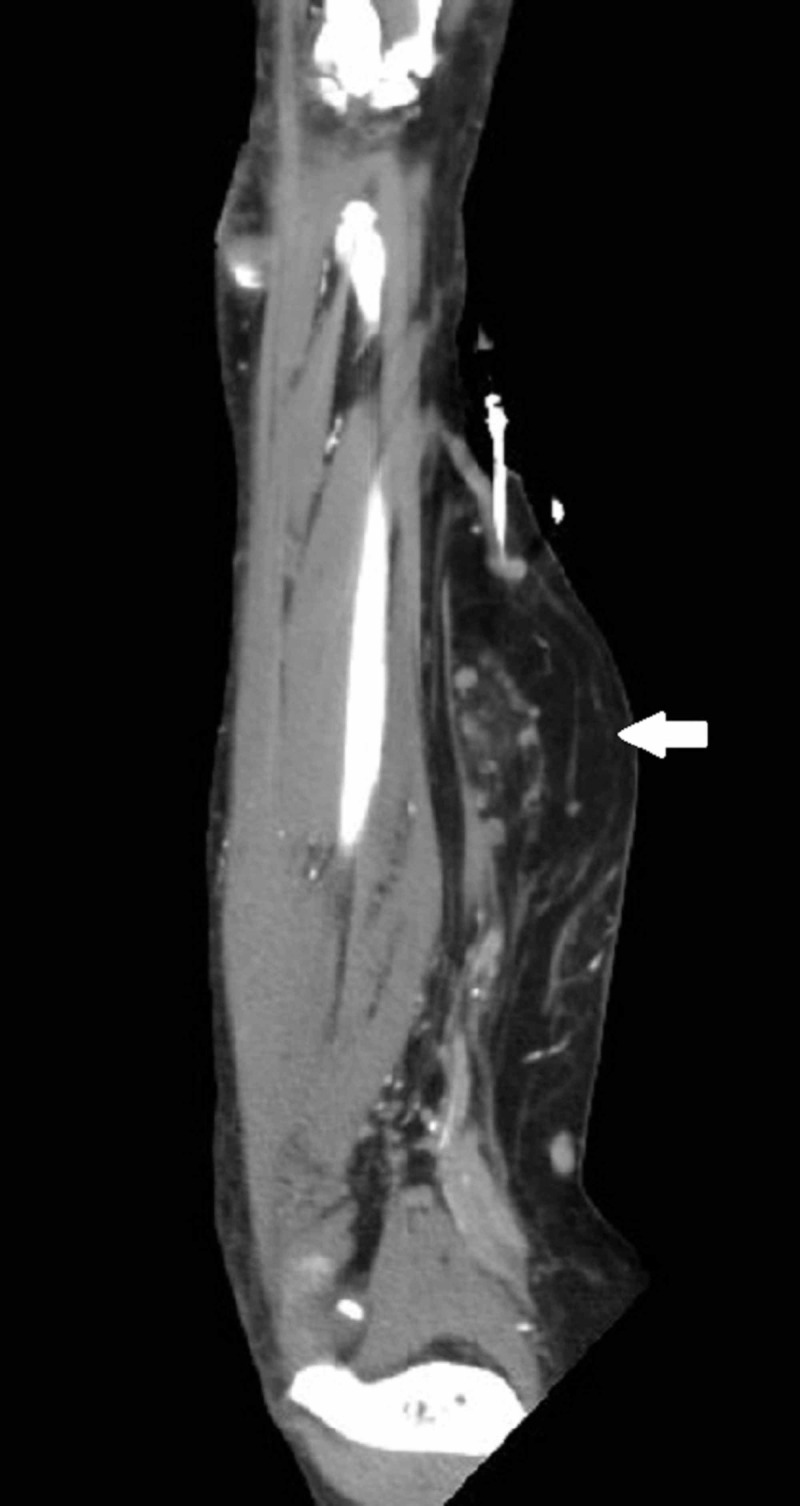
Coronal reformatted CT also showing a right forearm mass (thick white arrow) concerning for atypical lipomatous tumor or liposarcoma.
